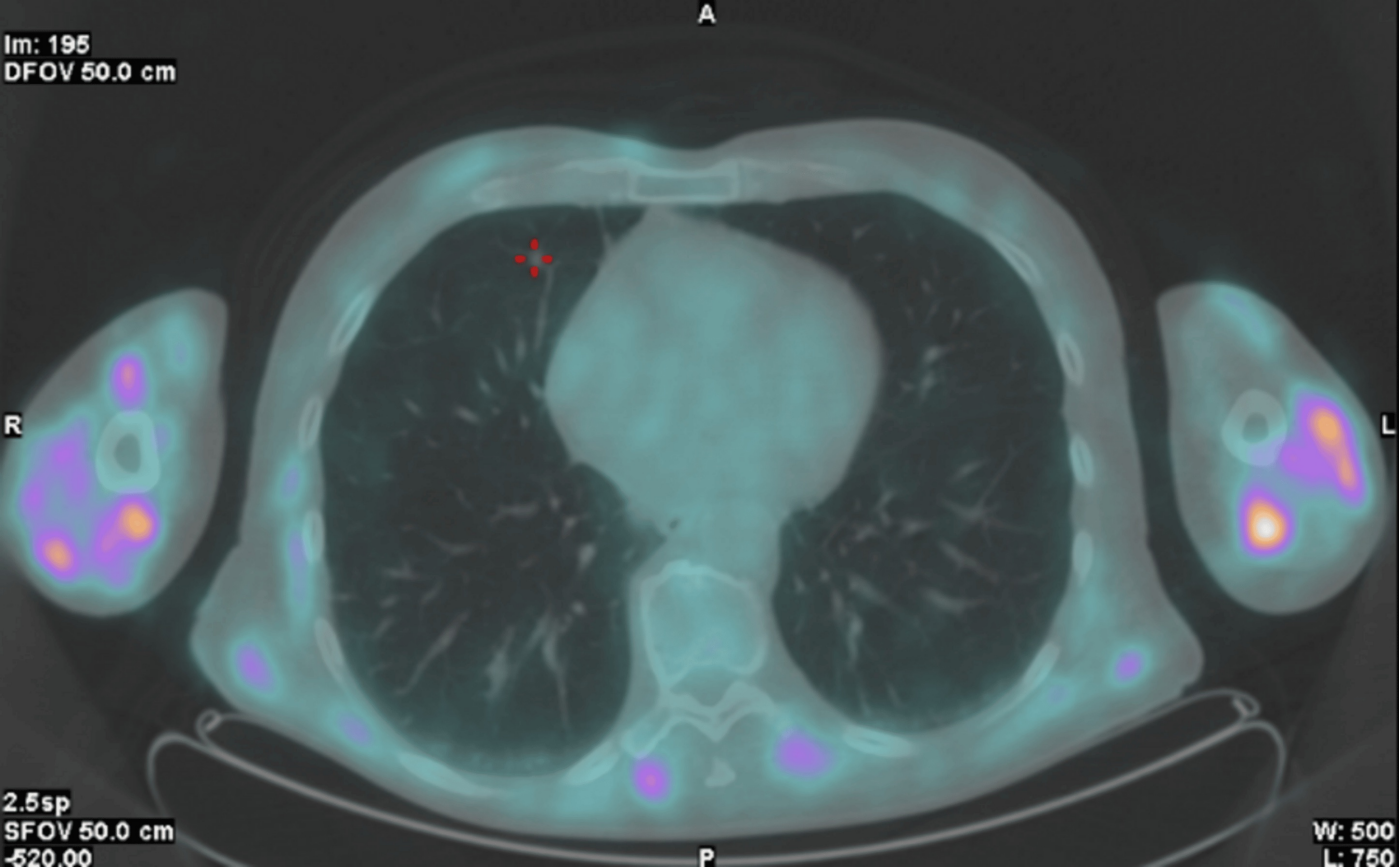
“Tiger man sign" on 18F-FDG PET/CT (axial fused image at the thoracic level), showing bilateral and symmetric hypermetabolic striated uptake in the skeletal muscles of the upper limbs, with no significant thoracic FDG-avid lesions. FDG: fluorodeoxyglucose.
